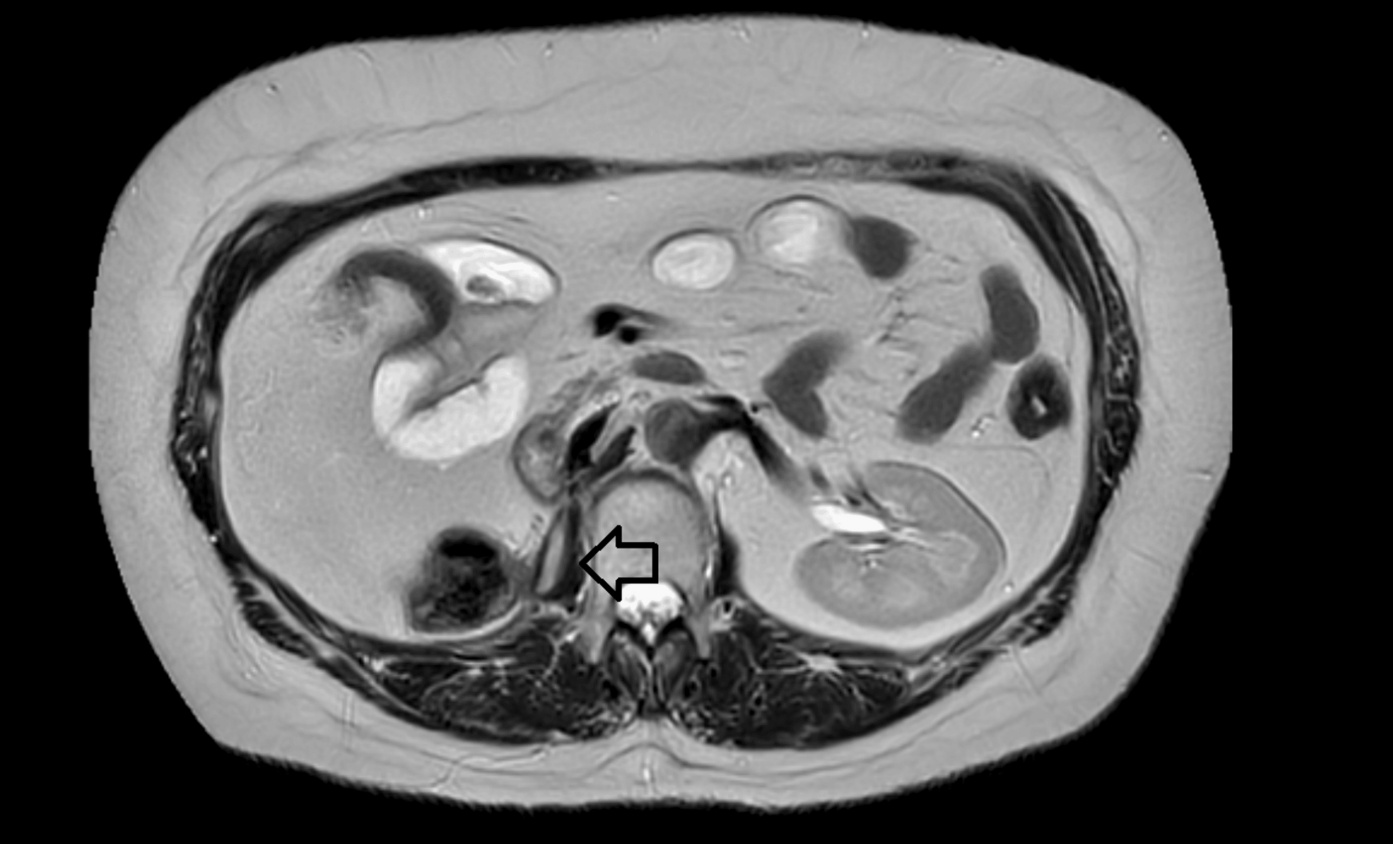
An axial slice of T2-weighted MRI image demonstrating disease recurrence (arrow) in the right psoas muscle with the lesion exhibiting hyperintense signal
